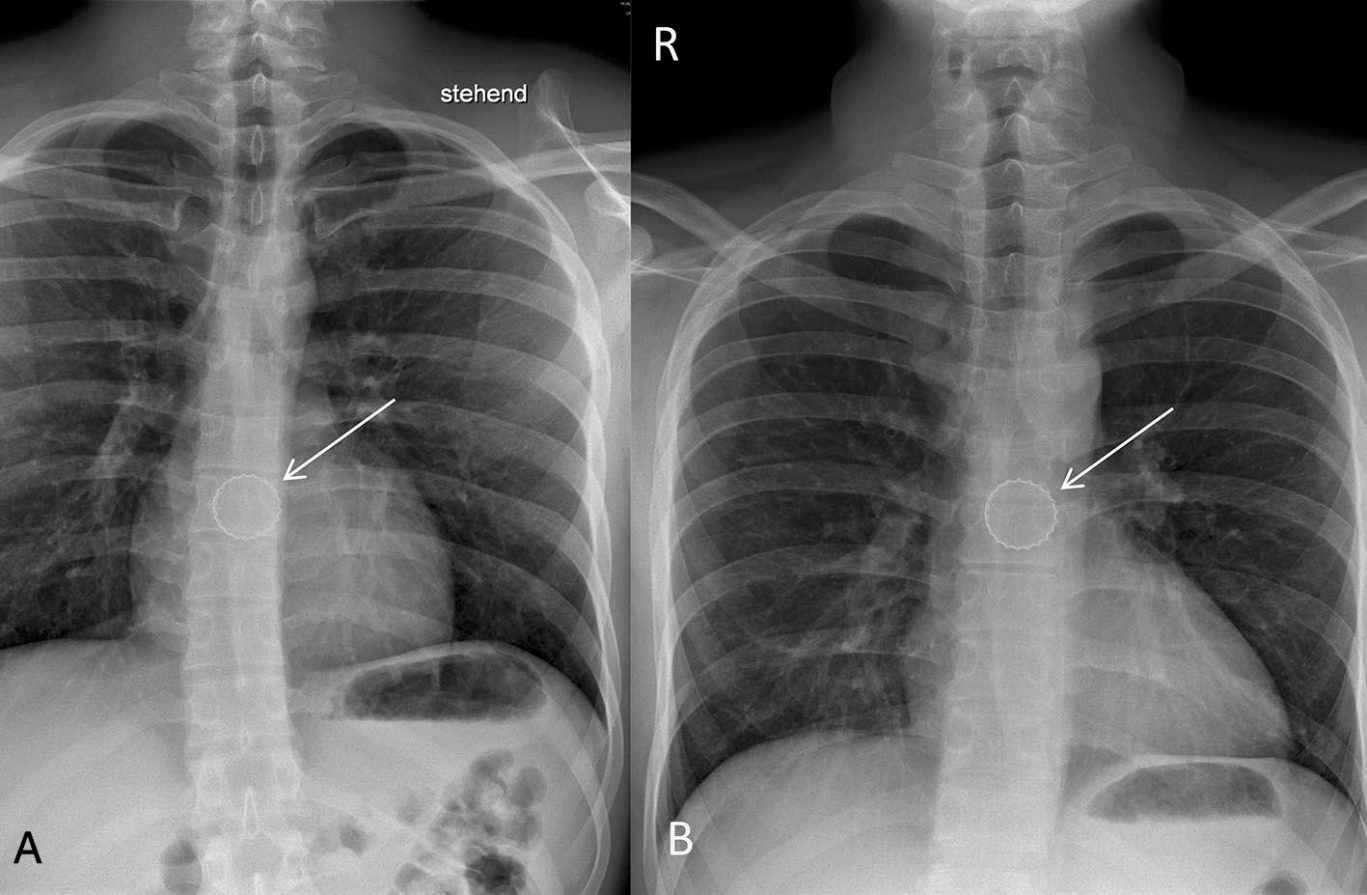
Lower esophageal narrowing. It lies at thoracic vertebrae near X/XI in the area of the esophageal hiatus shortly before the esophago-gastral transition. A: Posterior–anterior X-ray of the thorax (A). Bottlecap near thoracic vertebra VII (arrow). B: Posterior–anterior X-ray of the thorax (A). Bottlecap near thoracic vertebra VI/VII (arrow). *R* right; *L* Left; *a* anterior; *p* posterior
